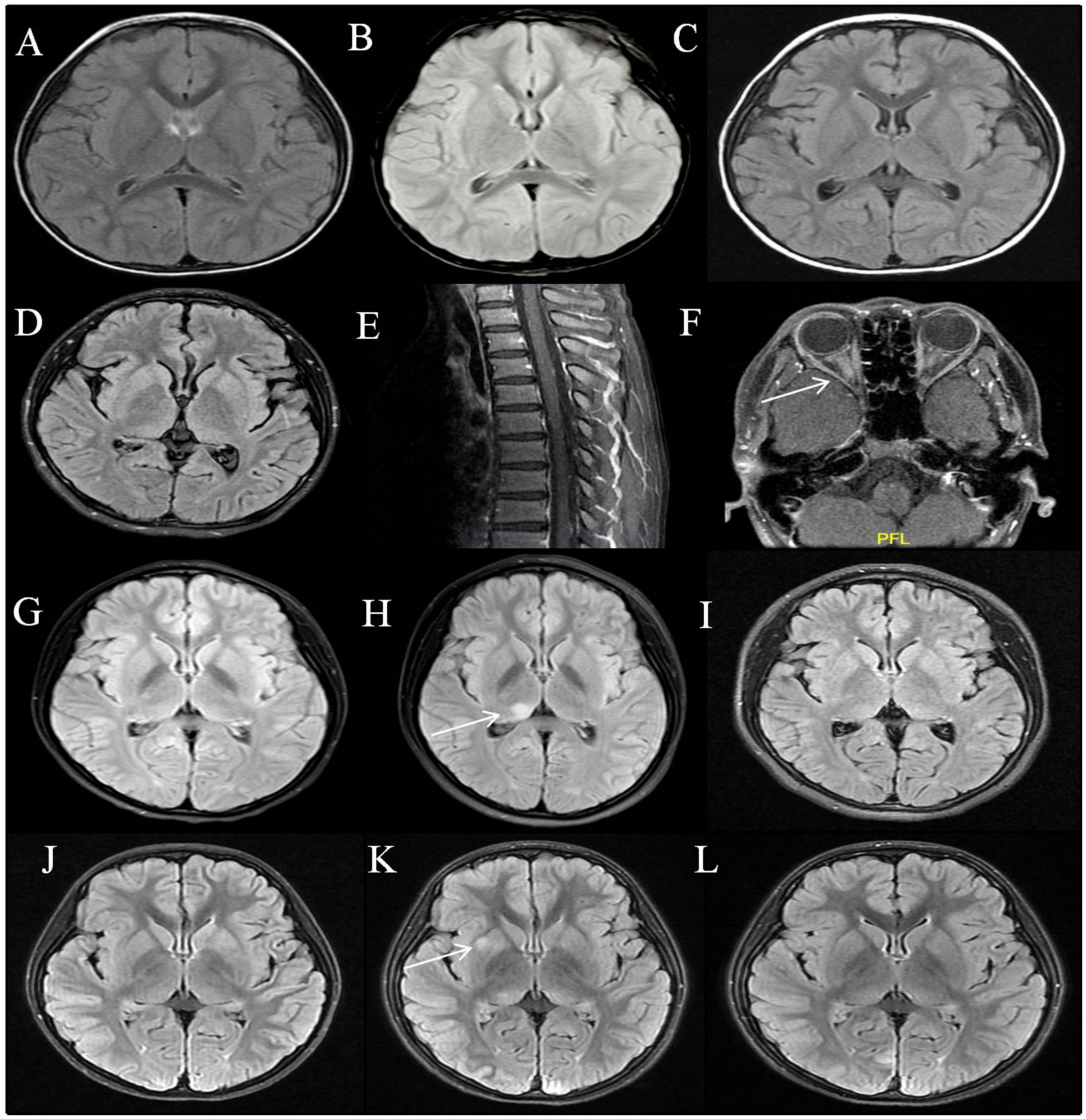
MRI features of MOG antibodies positive encephalitis. Patient 1: Normal brain MRI on the 11th, 20th, and 54th days from onset (A–C); Patient 16: Normal brain and spinal MRI at acute phase (D,E); Bilateral optic nerve enhancement on the 28th day after the onset (F). Patient 2: Normal brain MRI at the first attack (G); Six months after onset, a relapse occurred with T2 flair hyperintensity in the right thalamus (H); The lesion disappeared after immunotherapy (I). Patient 3: Normal brain MRI at acute phase (J); T2 flair hyperintensity in the right globus pallidus on follow-up scans 2 months later (K); The lesion almost disappeared 5 months after the onset of the disease without chronic immunotherapy (L).
